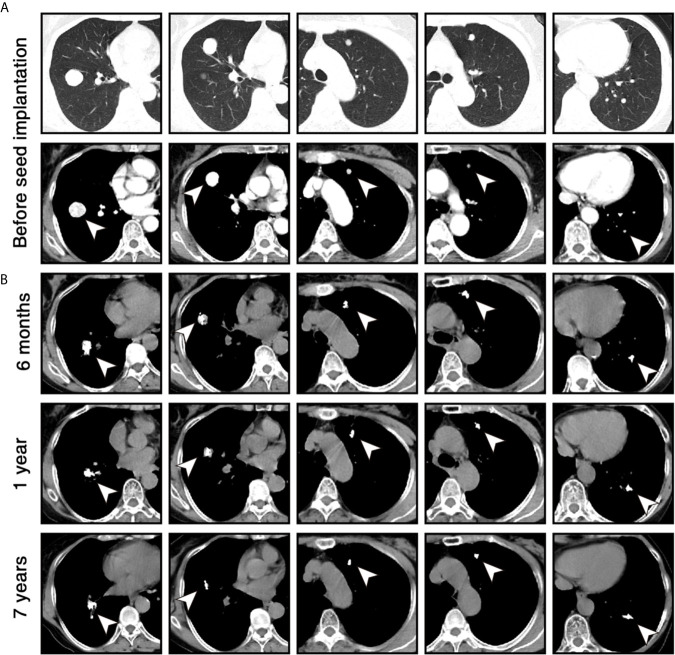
Chest computed tomography (CT) scans of the patient during the follow-up. (A) Before the ^125^I seed implantation, contrast-enhanced CT images in lung windows (upper) and mediastinal windows (lower) show 5 enhanced nodules (white arrowheads) in both lungs. (B) Six months, 1 year, and 7 years after ^125^I seed implantation, unenhanced CT images in mediastinal windows show that volume of lesions was significantly reduced within 1 year and remained stable for 7 years. High-density ^125^I seeds were located in the original sites of the lesions.
